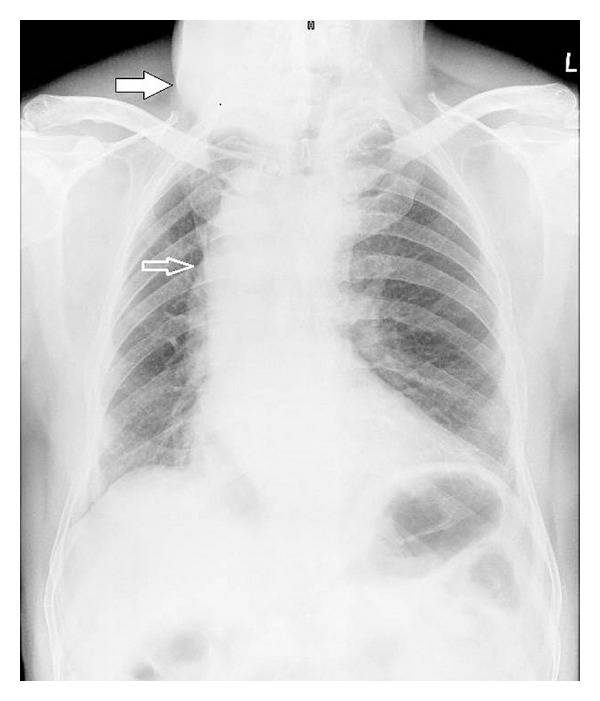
Frontal chest radiograph shows evidence of right-sided aortic arch (open arrow) and also a soft-tissue density in the neck representing thyromegaly (solid arrow).
